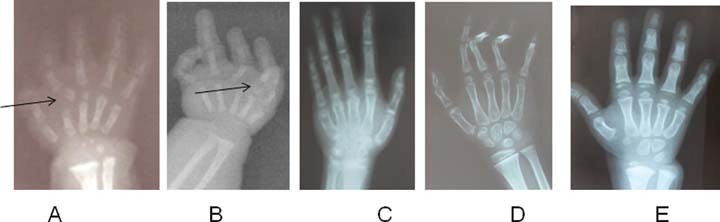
Hand X-rays of Desbuquois dysplasia. A: Patient 4 (p.Tyr178Leufs*4) had extra ossification centers distal to second and third metacarpals (2 months old). B: Patient 6 (p.Ala34Phefs*56 and p.Leu93Valfs*89) had extra ossification centers distal to the first and the fourth metacarpals associated with finger dislocations (fetal stage). C: patient 7 (p.Ser303Arg) presented similar hand anomalies compared to Kim variant with very short metacarpals and elongated phalanges (7 years old). D: patient 8 (c.−342+1G>A) showed finger dislocations, epiphyseal interphalangeal anomalies, and thumb digitalization without extra ossification center (2.5 years old). E: Desbuquois dysplasia type 2 patient (2 years old). Normal hand (4 years old). Advanced bone age can be observed in all patients.
